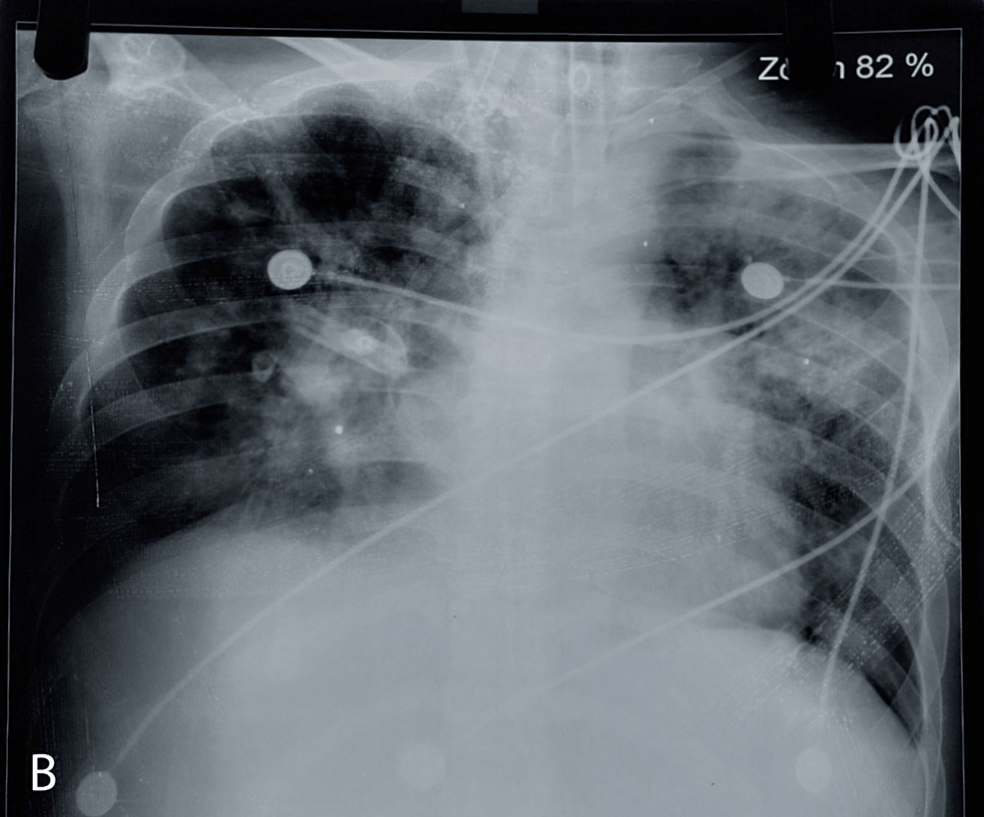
Last X-ray taken (day 28).
